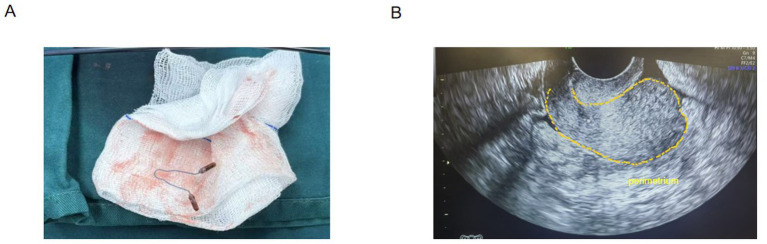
(A) Intact V-shaped IUD after removal *via* the uterine cavity. (B) Follow-up color Doppler ultrasound obtained 1 month postoperatively showing no abnormalities.
